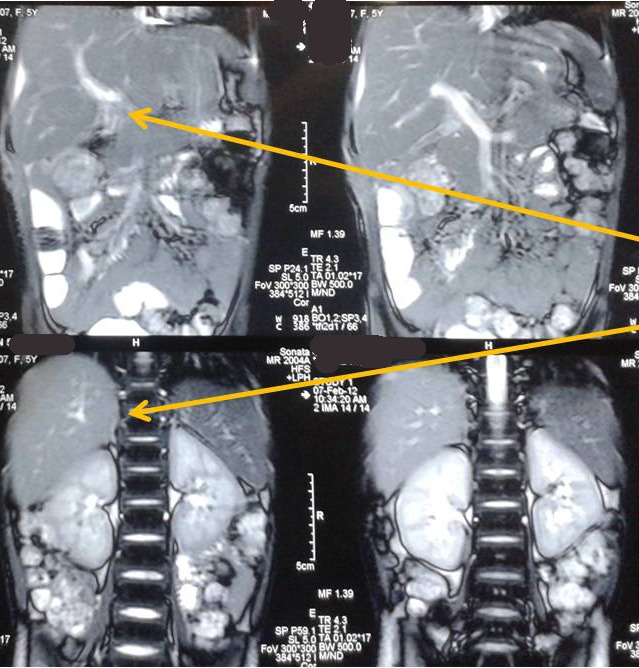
Figure 2:CT abdomen of the same patient at 6 months post injury, showing healed laceration (marked with arrow), with no intra-hepatic stasis/leak.
